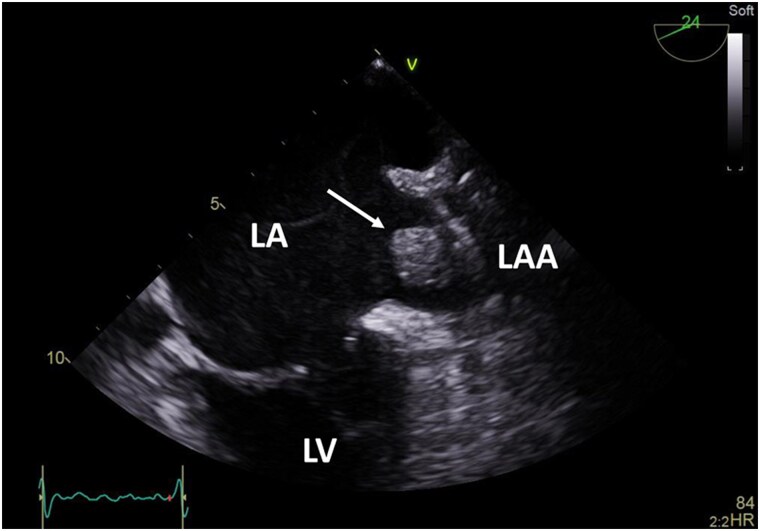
Transoesophageal echocardiography (TOE) of the left atrium. TOE demonstrating large thrombus (2.5 × 1.3 cm) in the left atrial appendage (LAA). LA, left atrium; LV, left ventricle.
